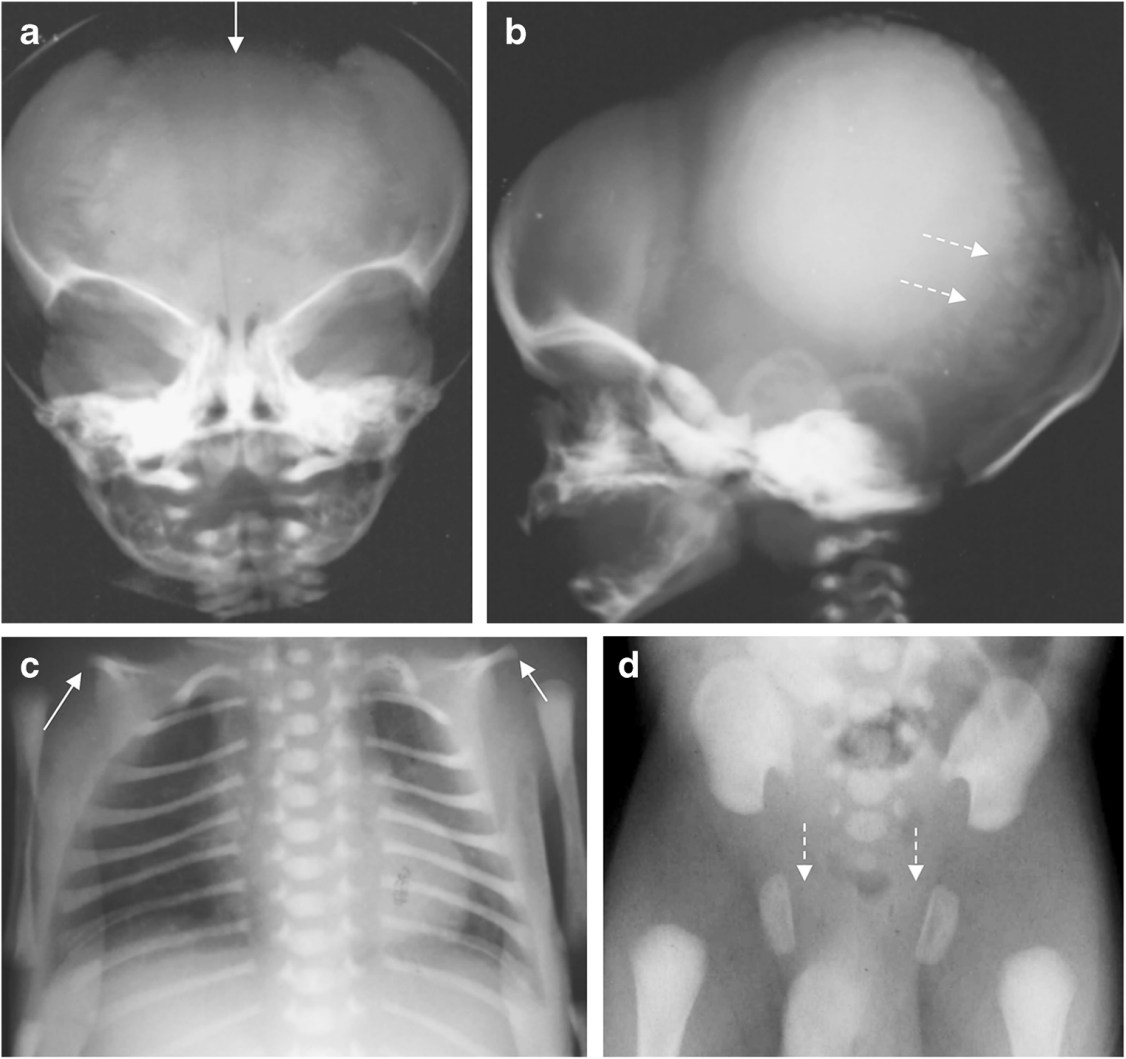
Radiographic features of cleidocranial dysplasia. a,b Radiographs of the skull in the anteroposterior view (a) and lateral view (b) show wide sutures and fontanelles (*arrow*) and multiple Wormian bones (*arrows*). c Anteroposterior chest radiograph shows hypoplastic clavicles (*arrows*). d Anteroposterior pelvic radiograph shows deficient ossification of the pubic bones (*arrows*). [Images reproduced with permission from Radcliffe Publishing (38), page 390, case 4, images 4a-b, and case 3, images 3b-c]
